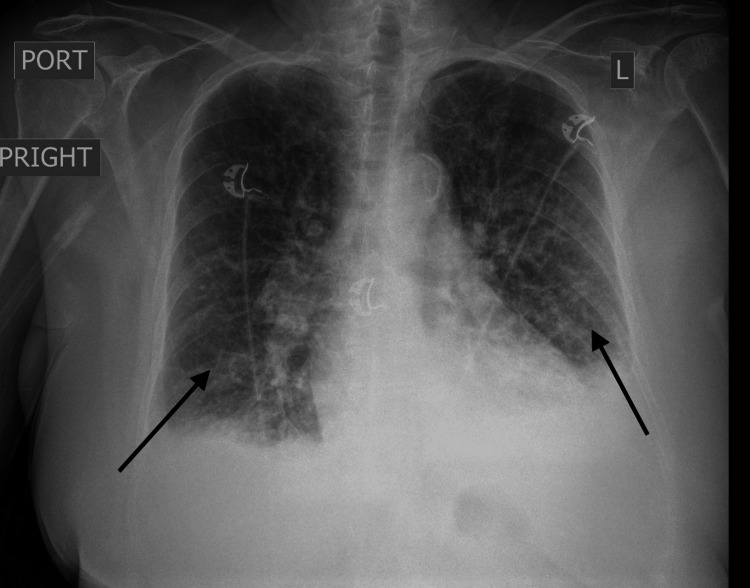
Chest X-ray demonstrating flash pulmonary edema with bilateral pleural effusions; arrows highlight key areas of pulmonary congestion and fluid accumulation
